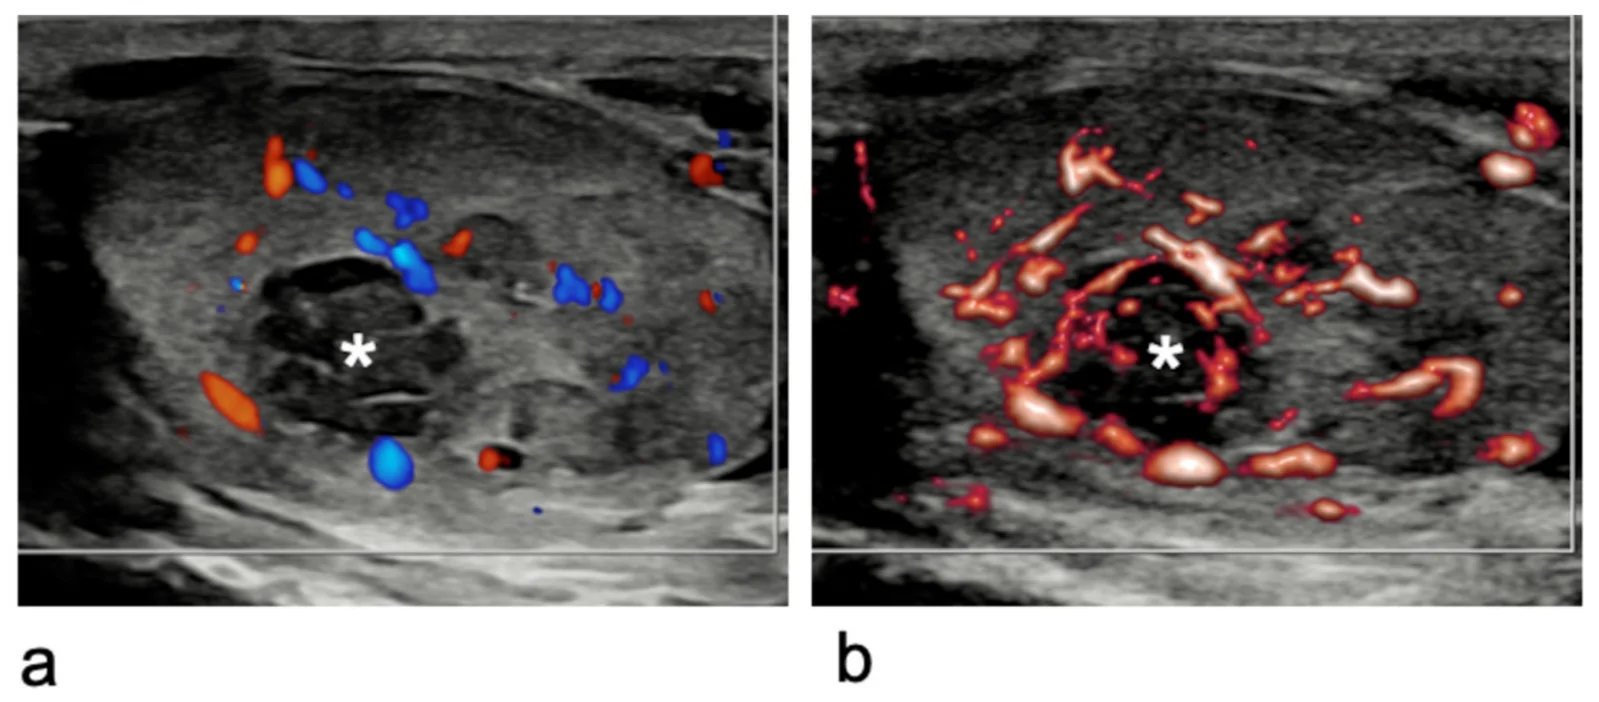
A 35-year-old man underwent ultrasonography for left testicular discomfort persisting for more than one week after minor trauma. (a,b) Ultrasound shows a hypoechoic lesion (asterisk) at the upper pole of the left testis, with no vascularity on colour Doppler imaging (a) but evident vascularity on microvascular imaging (b). At surgery, the lesion proved to be a seminoma.
